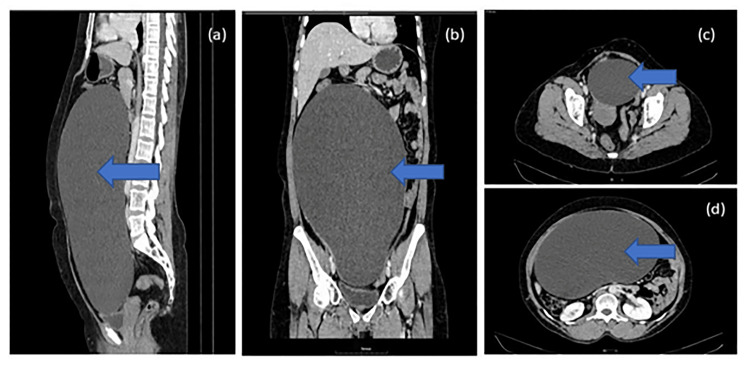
Contrast-enhanced CT (CECT) of abdomen and pelvis showing a) sagittal image, b) coronal image, c) transverse image pelvic region, d) transverse image upper abdomen
